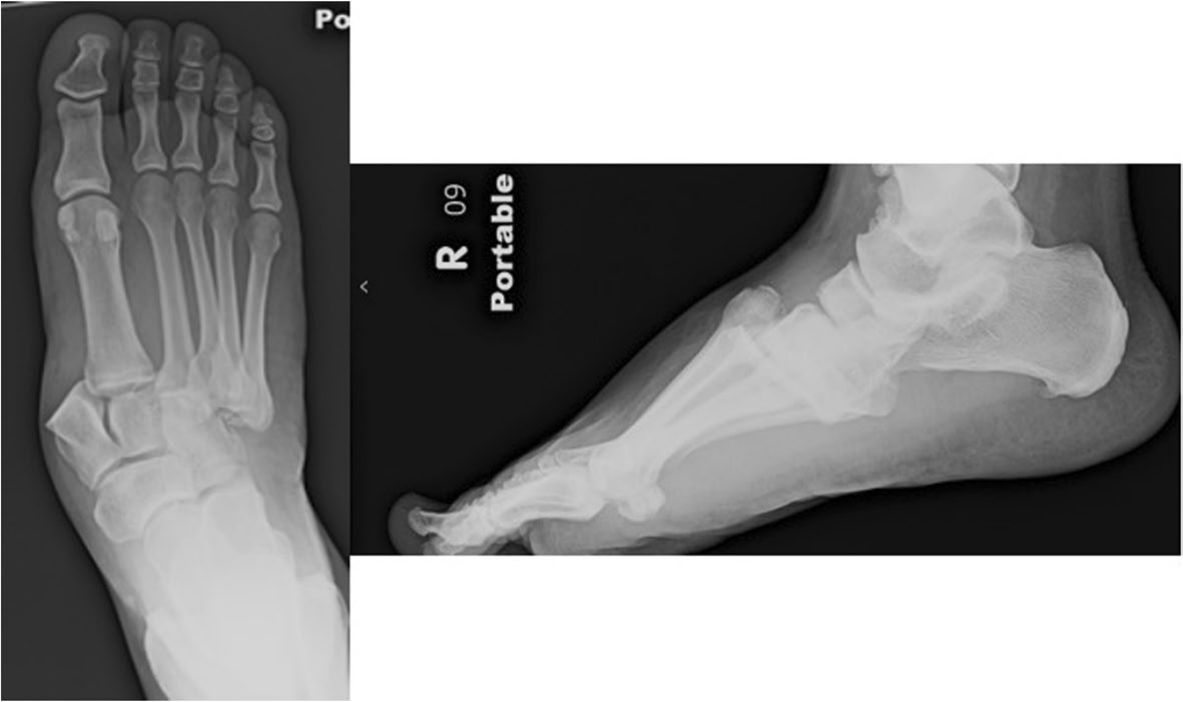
Anteroposterior and lateral injury radiographs of right foot demonstrating lateral displacement of the second metatarsal in a displaced Lisfranc joint fracture-dislocation, typical of the patients treated in this series
